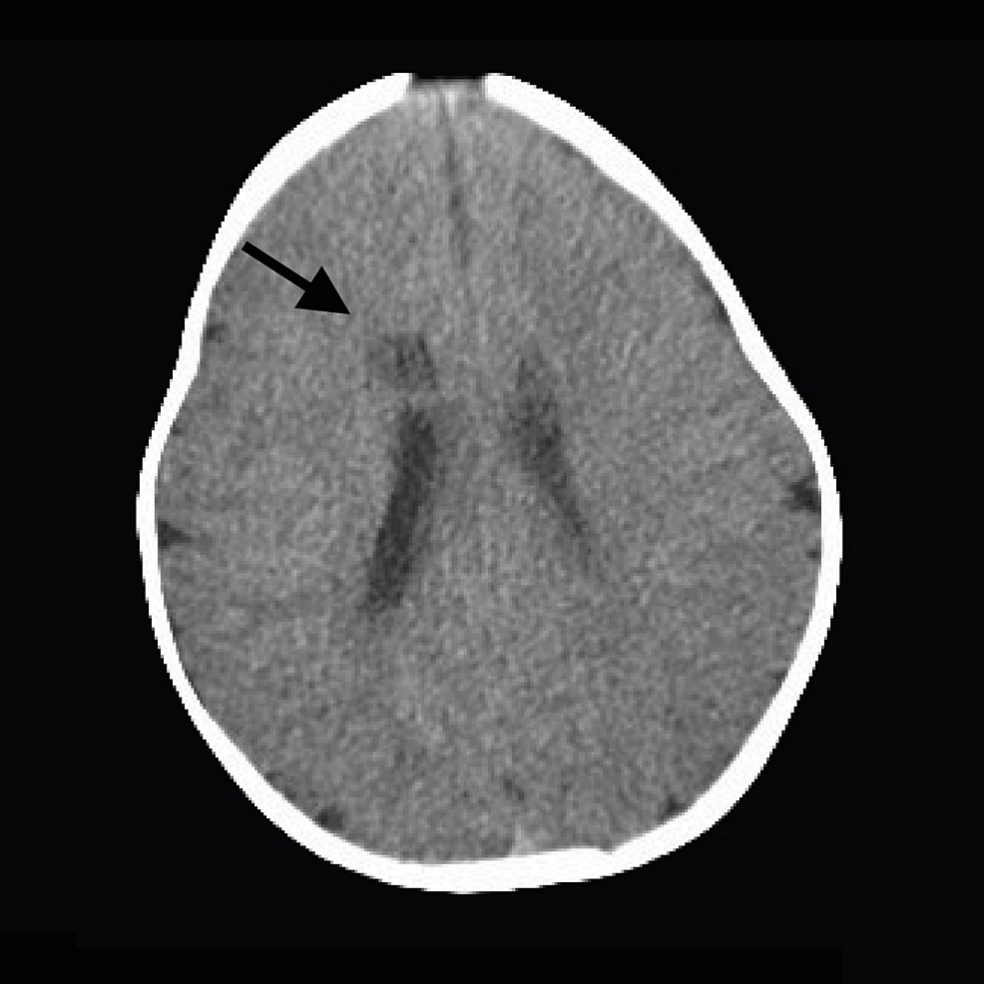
Axial plane of head CT without contrast. Cavernous malformation can be seen (black arrow).
